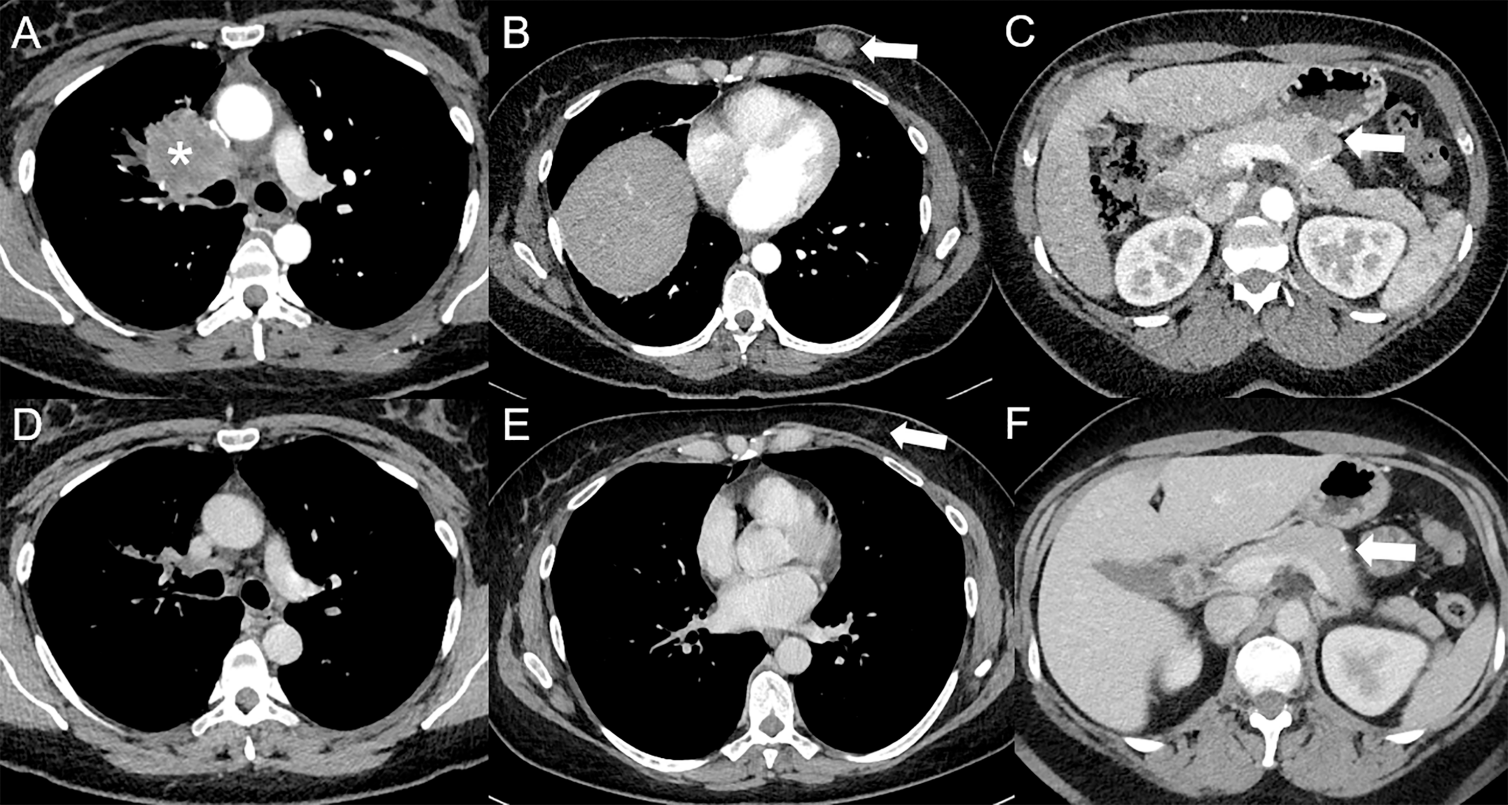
Complete response following immunotherapy in a 41-year-old female diagnosed in February 2016 with stage IV poorly differentiated lung adenocarcinoma in right upper lobe [asterisk *, (A)], with hypodense subcutaneous (arrow, (B)), and pancreatic [arrow, (C)] metastases visible on baseline contrast-enhanced CT at the arterial phase (A–C), treated with durvalumab (anti-PD-L1) and tremelimumab (anti-CTLA-4), followed by durvalumab maintenance. Since May 2017, a complete response was obtained. In April 2022, a complete response was still observed at the primary tumor (D) and subcutaneous [arrow, (E)] sites, whereas only a squealer calcification [arrow, (F)] can be seen at the pancreatic site on contrast-enhanced CT at the portal venous phase (D–F).
